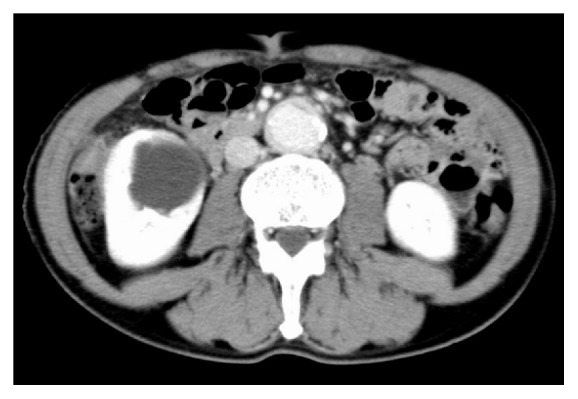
CT scan when the patient was first diagnosed with IgG4-RD with peritoneal fibrosis and periaortitis. CT, computed tomography; IgG4-RD, IgG4-related disease.
